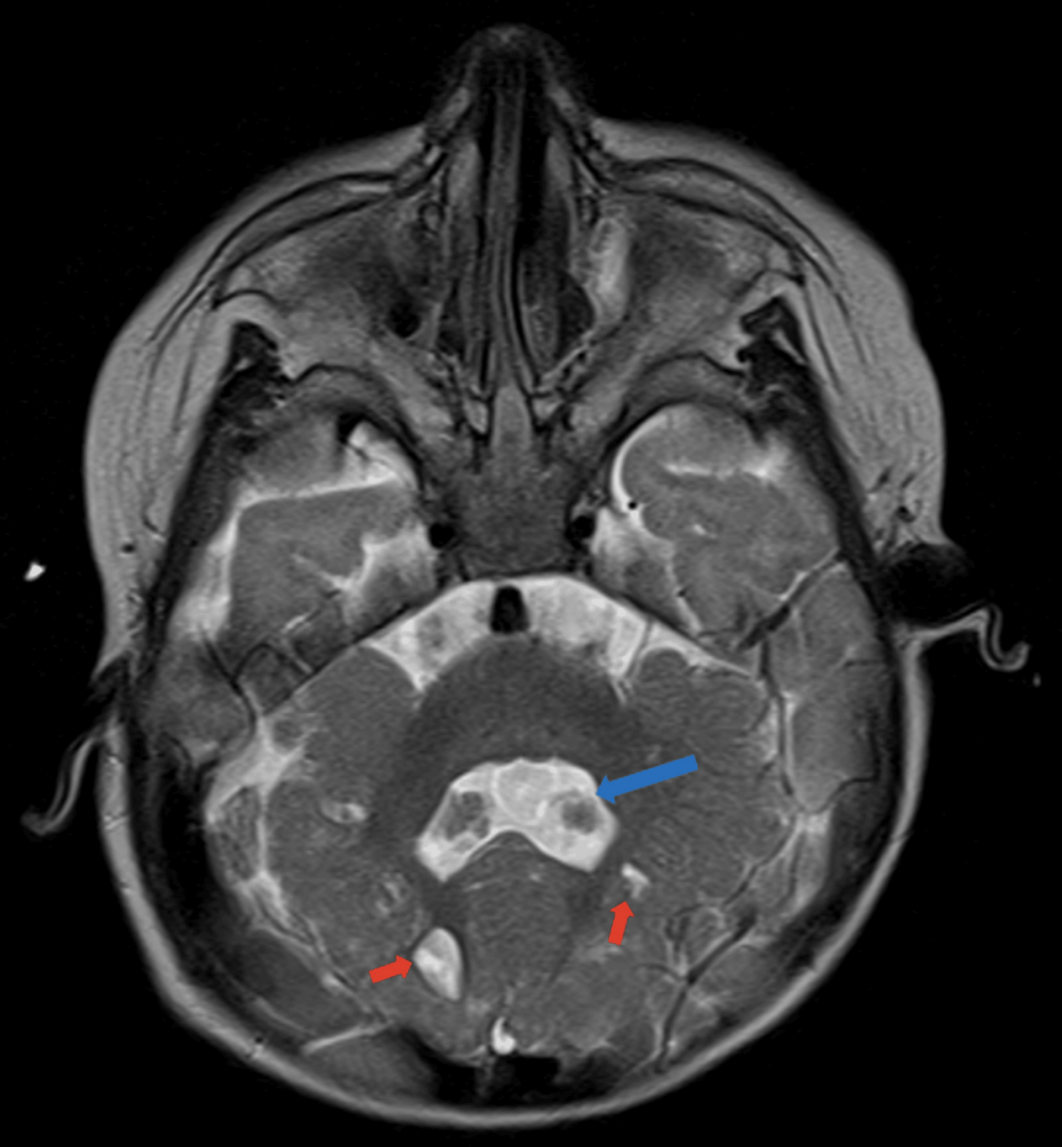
An axial section of the T2 brain MRI scan of case 2. Red arrows: cerebellar parenchymal cysts. Blue arrow: dilated fourth ventricle.
